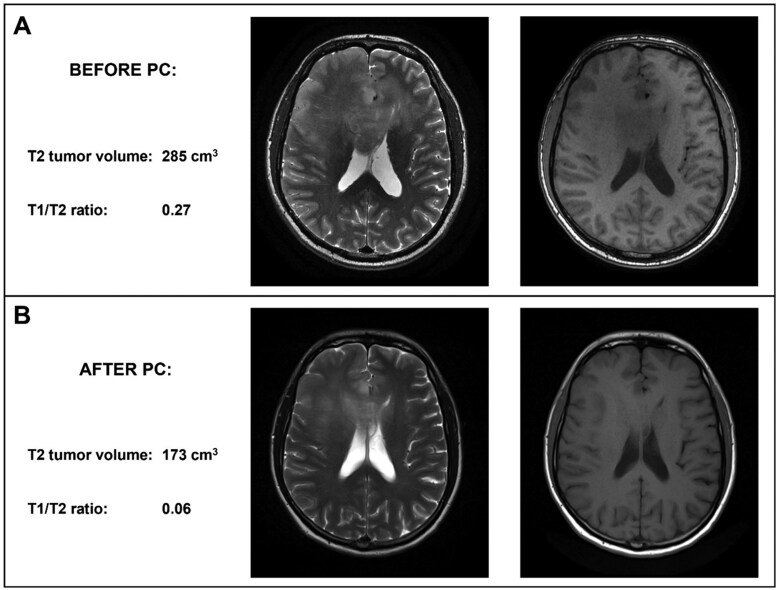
T2- and T1-weighted MRI scans of patients diagnosed with oligodendroglioma prior to PC polychemotherapy (A) and after discontinuation (B). MRI, magnetic resonance imaging; PC, procarbazine and CCNU (lomustine).
